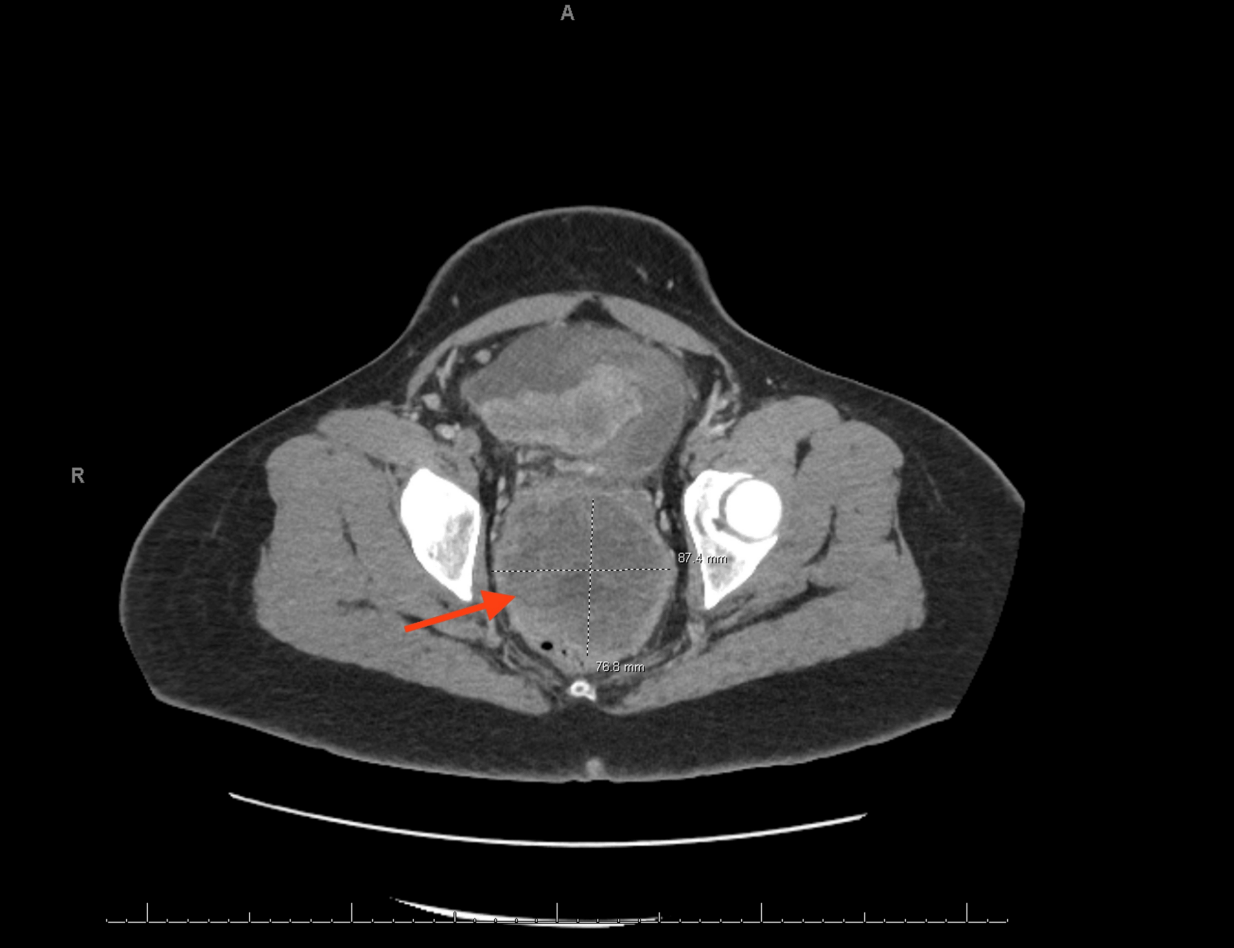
CT of the pelvis with contrast. The image displays the chest CT scan with contrast, and the arrow indicates the pelvic mass.
